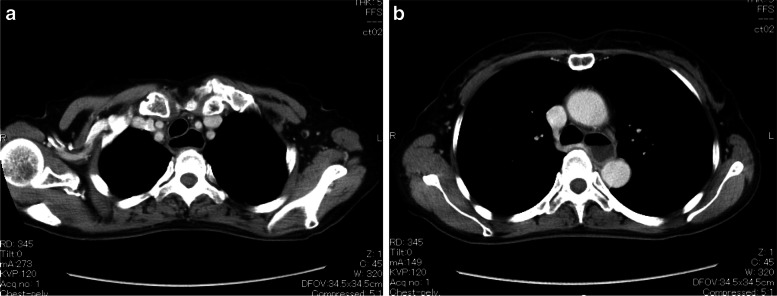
Preoperative thoracic computed tomography showing fluid retention in the dilated esophagus. a Upper thoracic region. b Middle thoracic region
